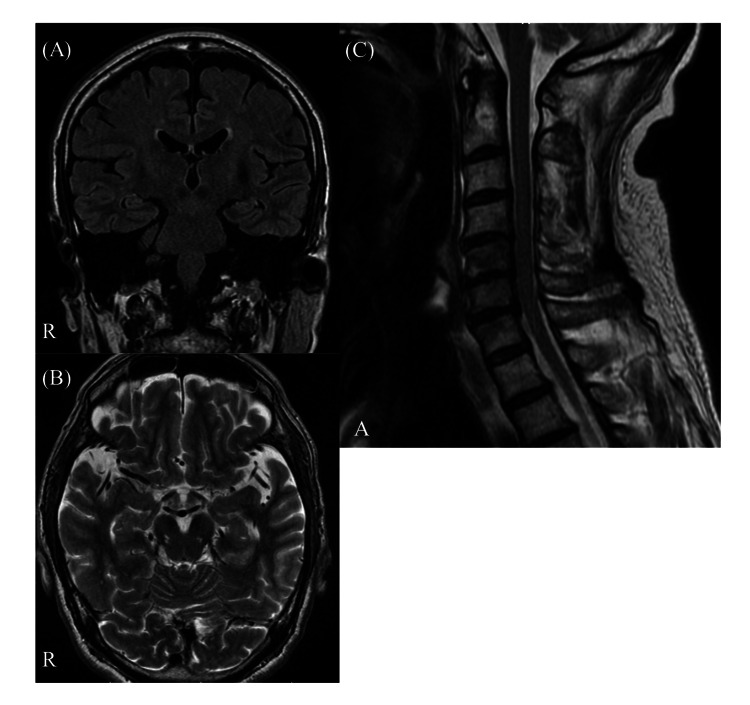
MRI of the brain (A, B) and cervical spine (C) without contrast agent on admission. (A) Coronal FLAIR MRI of the brain without contrast agent. (B) Axial T2-weighted MRI of the brain at the midbrain and hippocampus level. (C) Sagittal T2-weighted MRI of the cervical spine. All these images show no significant abnormal findings. MRI, magnetic resonance images; FLAIR, fluid-attenuated inversion-recovery
